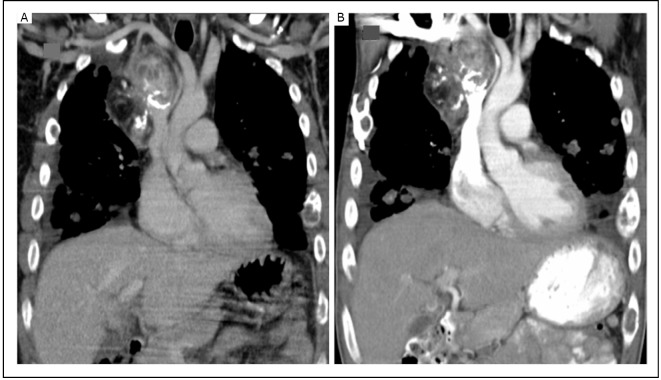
A: CT scan (coronal view) showing a mass in the anterior and superior mediastinum containing a solid-enhancing component, coarse calcification, and fat. The mass involves the superior vena cava and right brachiocephalic vein. B: CT scan after 6 months showing stable appearance.
